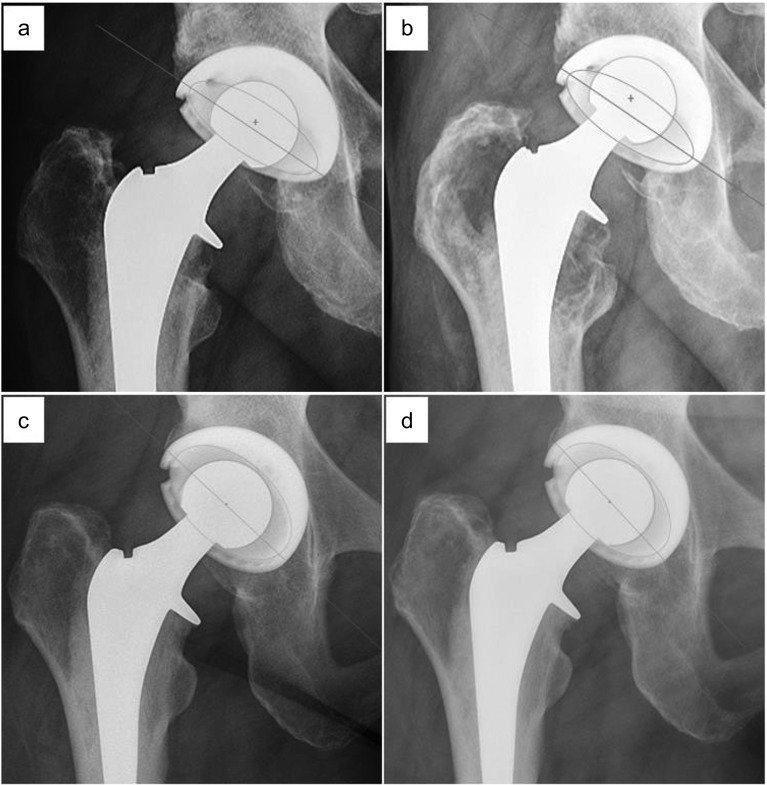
(a) Postoperative and (b) 11.31 year follow-up radiographs of a 28 mm cobalt chrome head on UHMWPE Reflection polyethylene liner. Linear penetration of the head was measured to be 5.581 mm, with observed femoral osteolysis in Gruen zones 1 and 7 (white arrowheads). (c) Postoperative and (d) 10.53 year follow-up radiographs of a 28 mm cobalt chrome head on XLPE Reflection polyethylene liner. Linear penetration of the head was measured to be 0.335 mm, with no observed osteolysis. UHMWPE: ultra-high molecular weight polyethylene, XLPE: highly cross-linked polyethylene.
